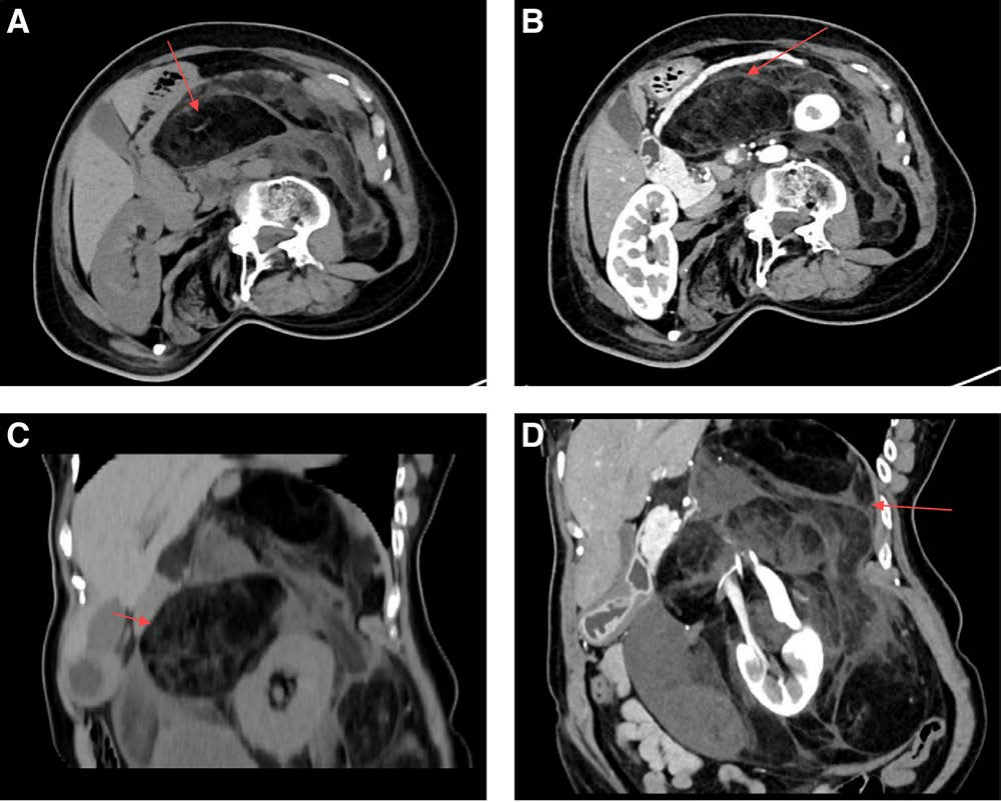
(A) Axial CT scan demonstrates a large retroperitoneal mass with heterogeneous density, causing displacement of adjacent structures. (B) Contrast-enhanced CT reveals the mass with mild delayed enhancement, further suggesting a malignant process. (C and D) The liposarcoma can be seen encircling the kidney on both non-contrast and contrast-enhanced CT scans. (D) heterogeneous enhancement of the liposarcoma tissue is observed.
